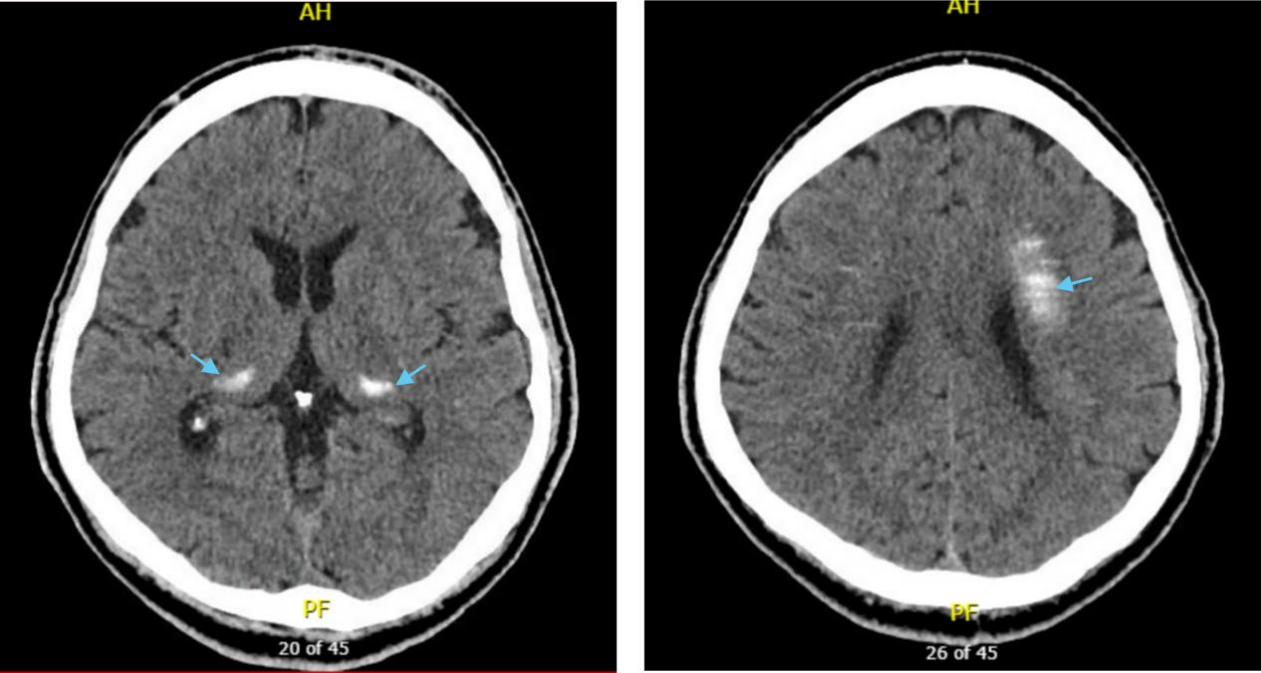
Noncontrast axial computed tomography images of the head in 2013 showed calcifications in the bilateral basal ganglia, including the globus pallidus, posterior aspect of thalami, dentate nuclei, and corona radiata.
